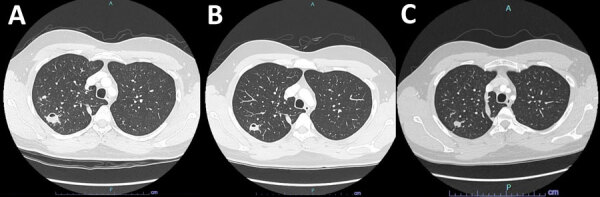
Computed tomography (CT) scans for reported case of *Tsukamurella tyrosinosolvens* infection in 25-year-old immunocompetent man. A) Initial CT showing 25-mm cavitating right apical lesion. B) Repeat CT after 6 months of treatment, indicating interval cavitary lesion reduction to 10 mm and resolution of the second smaller cavitation seen on original CT. C) Final CT 6 months after treatment cessation, indicating further cavity resolution without evidence of infection recurrence.
